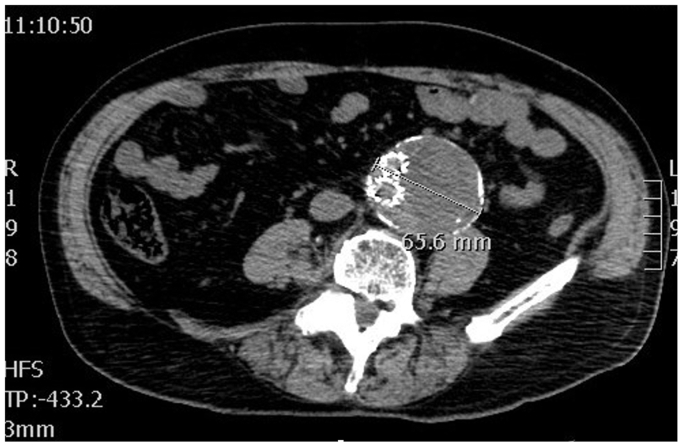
Computed tomography (CT) control scan after 1 year.
